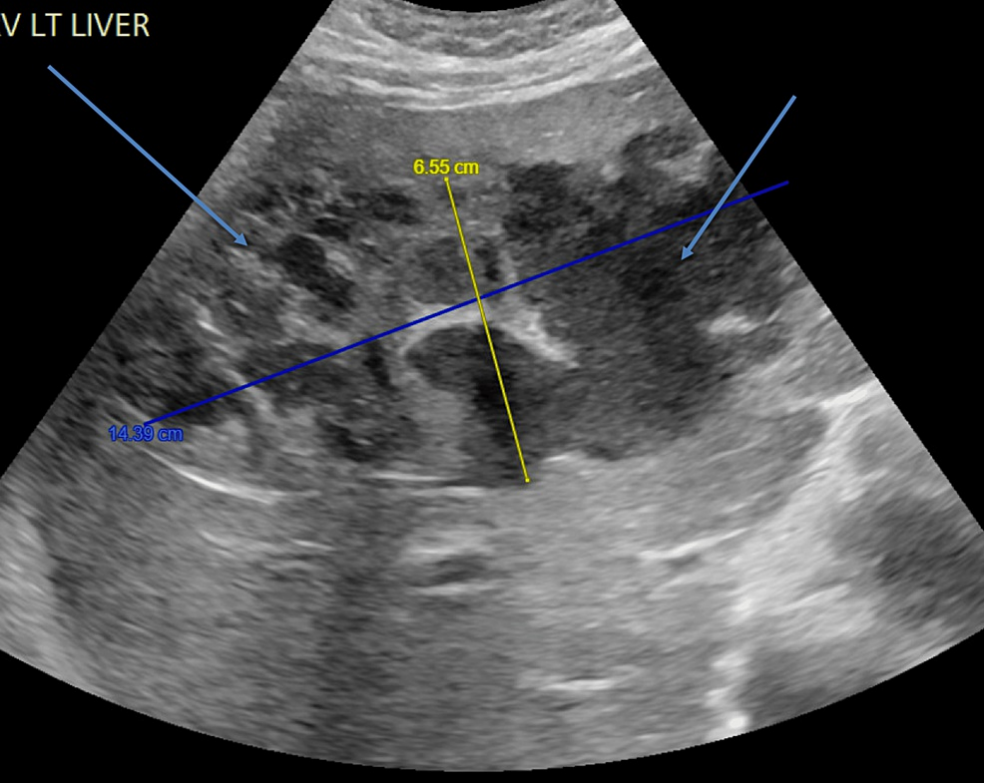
Ultrasound of the abdomen. A complex cystic mass measuring 14.39 cm x 6.55 cm in the right lobe of the liver suggests the presence of a liver abscess.
